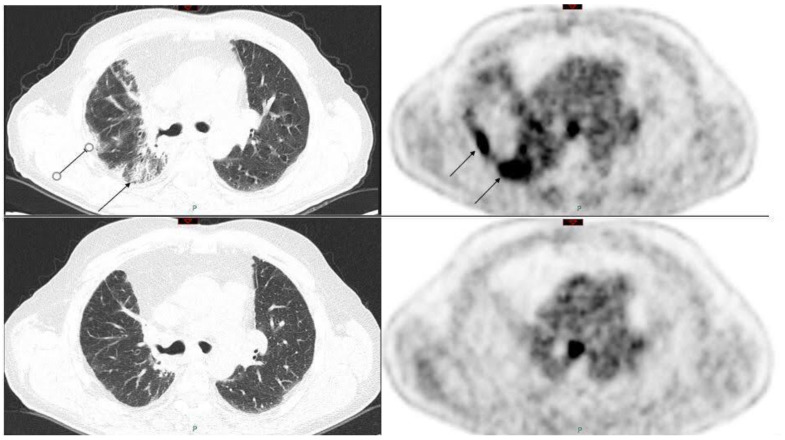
(Upper row): pre-therapy 18F-FDG PET/CT of a patient affected by lung cancer in treatment with atezolimumab, showing some areas of increased uptake of the radiopharmaceutical corresponding to mixed ground-glass and crazy paving lung CT alterations (black arrows). (Lower row): in PET/CT three months later, after atezolimumab suspension, no more areas of uptake are present, therefore indicative of an immune-related pneumonia.
